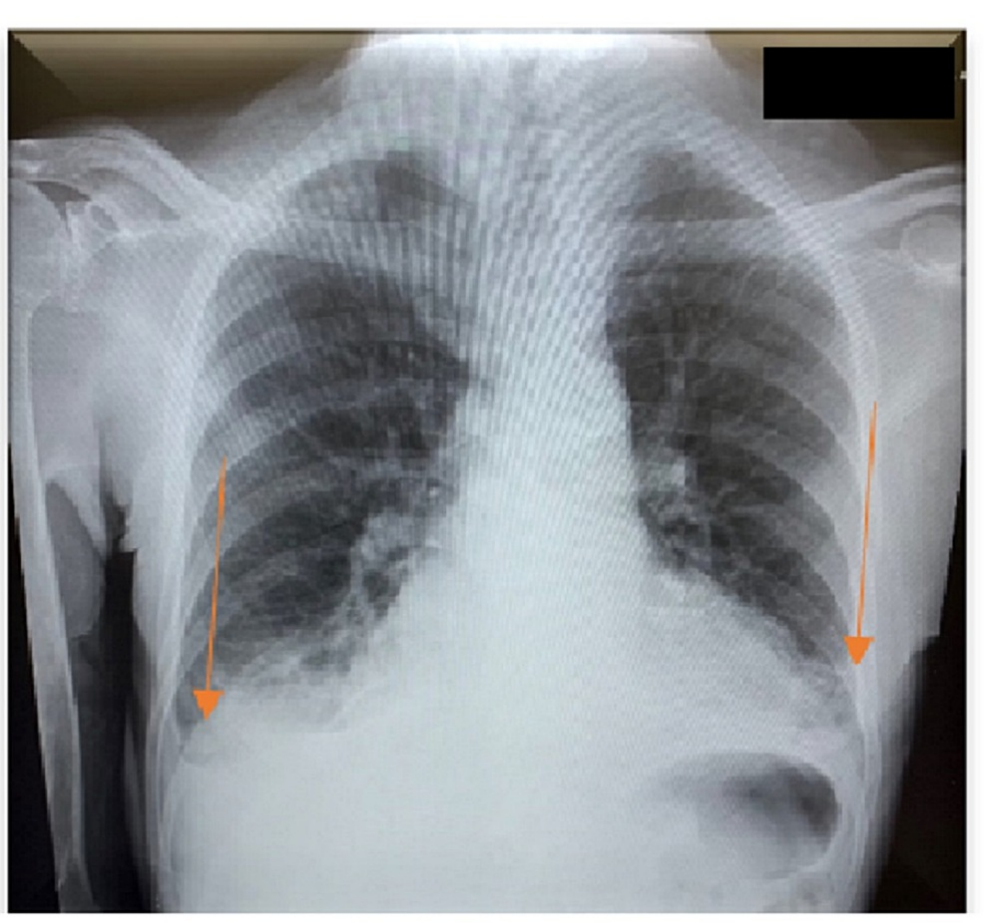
Chest X-ray with bilateral basilar pleural opacities.
